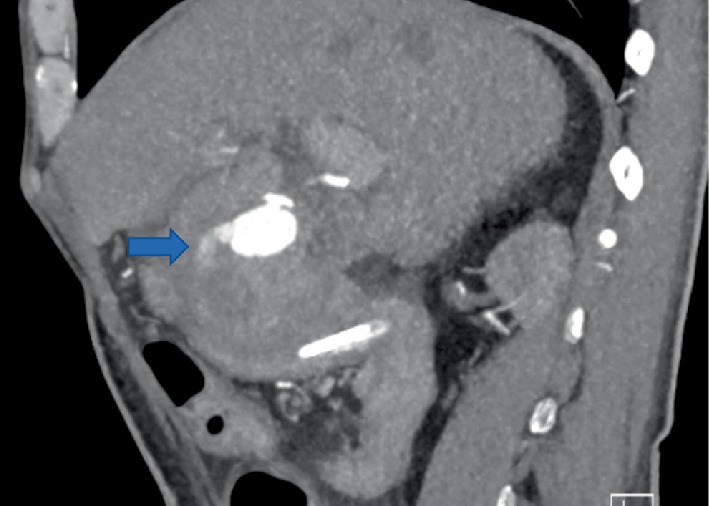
CTA case 1, performed POD #85 showing hepatic artery pseudoaneurysm with active extravasation (arrow).
